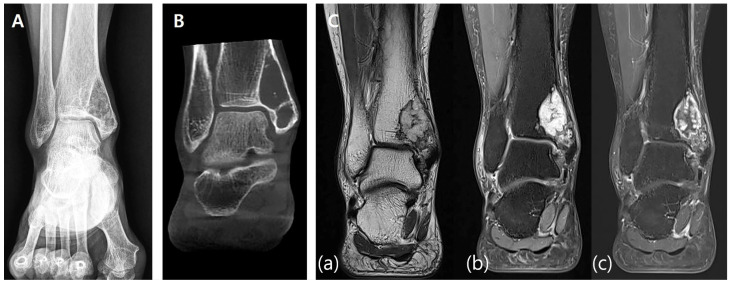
Preoperative imaging. (A) Preoperative X-ray demonstrating a lytic lesion with well-defined margins in the tibia. (B) Preoperative CT scan showing the lesion’s cortical involvement and surrounding bone structure integrity. (C) Preoperative MRI. (a) T1-weighted image displaying a hypointense lesion within the tibia. (b) T2-weighted image showing heterogeneous hyperintensity with internal low-signal regions. (c) Contrast-enhanced T1-weighted image highlighting the lesion’s vascular characteristics.
